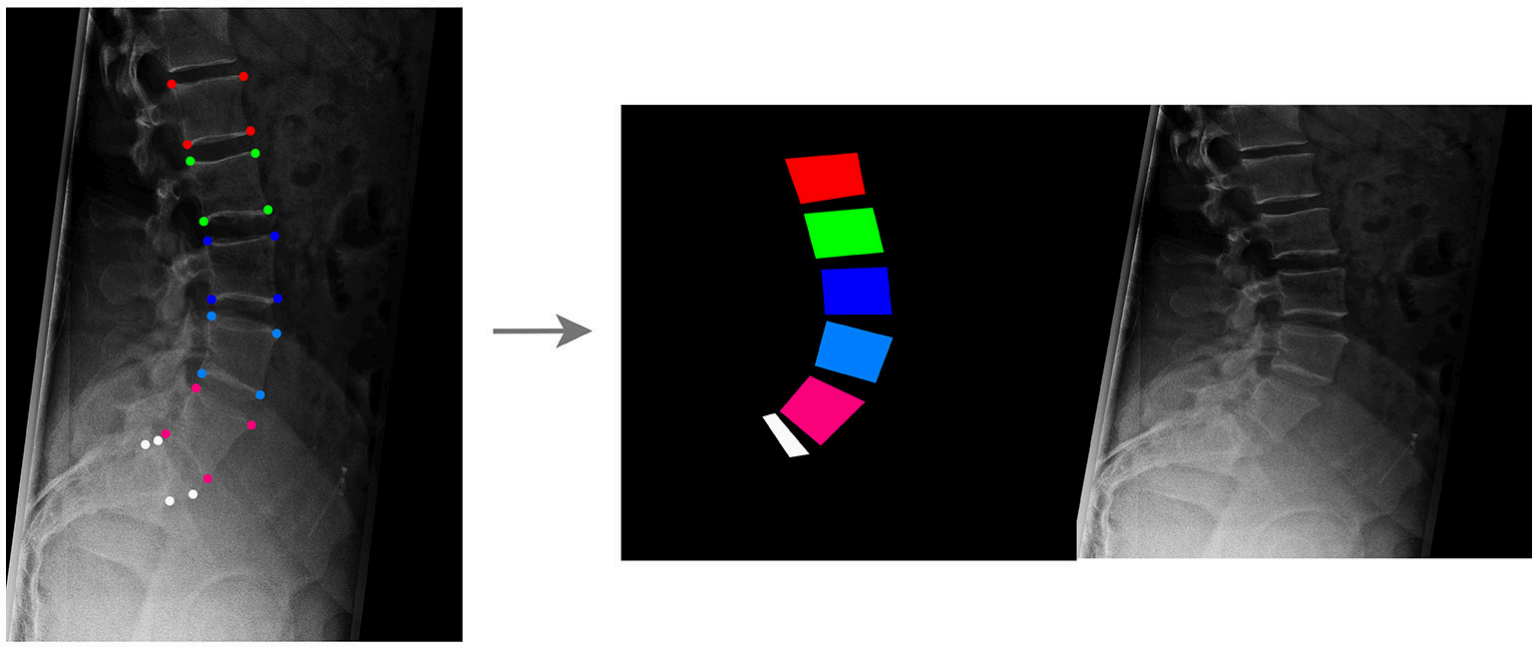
Creation of the training dataset for the GANs aimed to generate synthetic planar X-rays from labels. Vertebral corners, from L1 to L5 and for the upper aspect of the sacrum, are manually identified in each image (Left); based on the coordinates of the points, an image containing the label data on the left and the target radiograph on the right is generated (Right).
